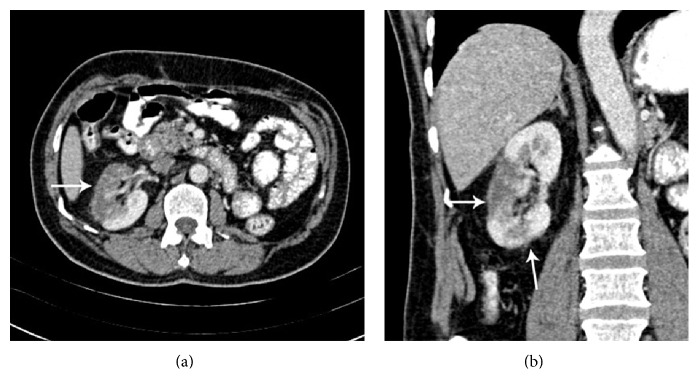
Findings: axial (a) and coronal (b) image of CT angiography in venous phase showing nonenhancing wedge shaped areas at mid and lower pole (arrows) of right kidney suggestive of infarcts.
